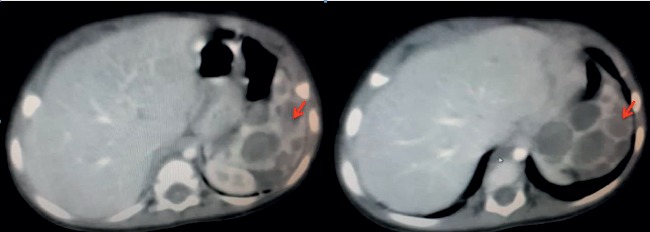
CT scan of the abdomen that shows the lymphangioma cysts (red arrow) of the spleen.
